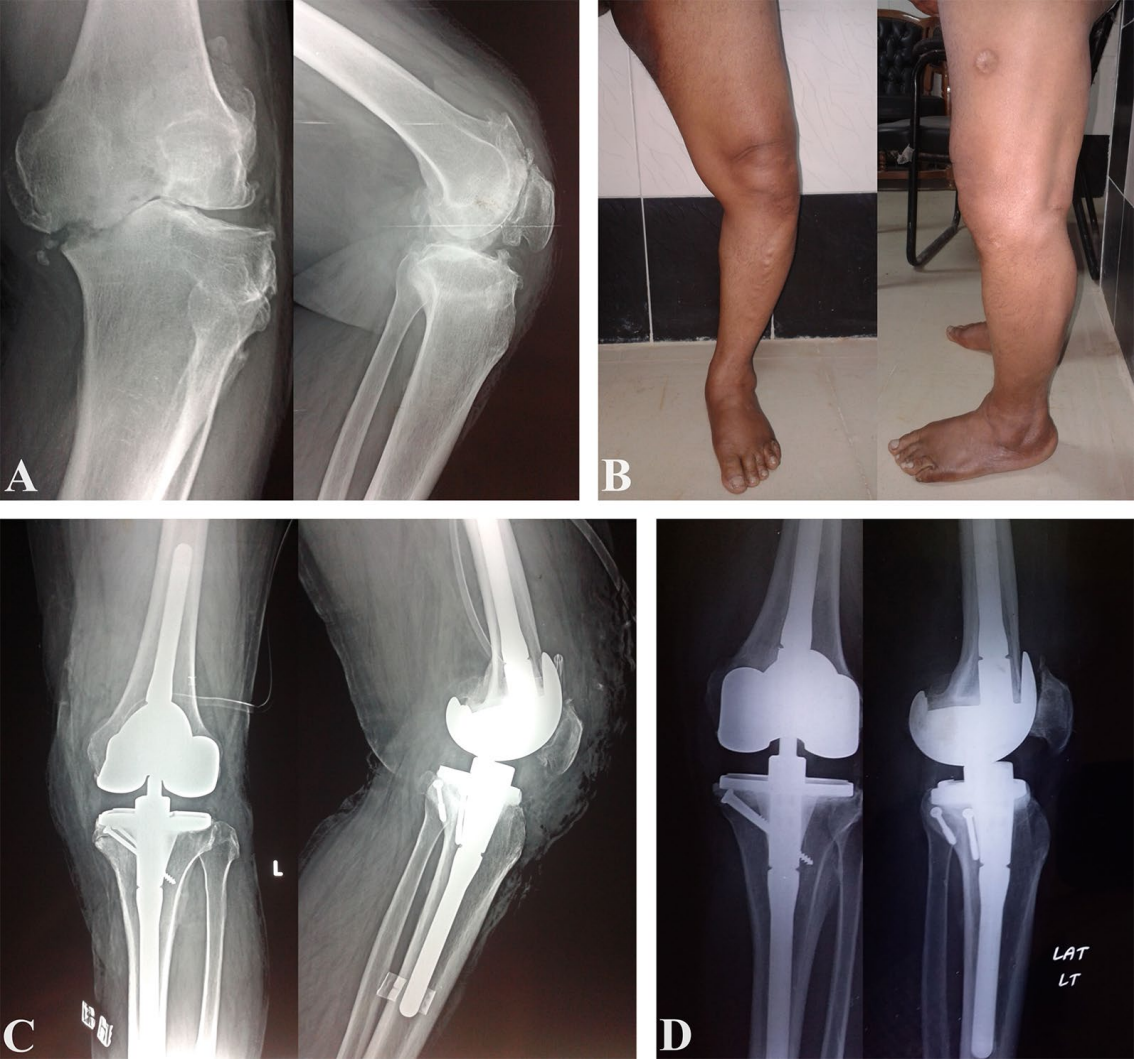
A 58-year-old male with advanced left knee OA. A Preoperative X-rays showing advanced OA and the medial proximal tibial defect. B Preoperative standing photographs showing varus deformity. C Postoperative X-rays after TKA using LCCK and reconstruction of the defect using structural autograft block fixed with two screws. D Three-year follow-up X-rays showing complete union of the graft
